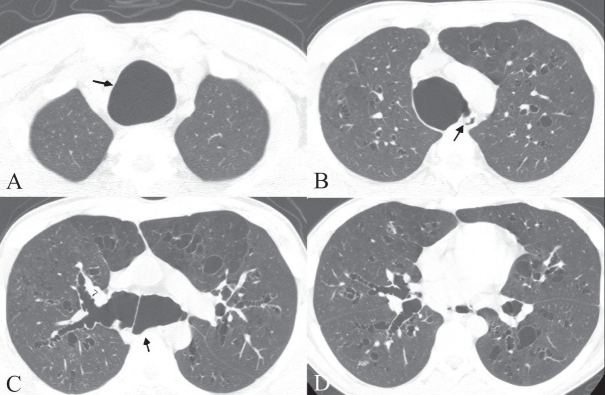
Axial high-resolution CT images show marked dilatation of the trachea (arrow in A), posterior tracheal diverticula (arrow in B), dilatation of the main bronchi (arrows in C), multiple diverticula (arrowheads in C), and bilateral cystic bronchiectasis (D)
